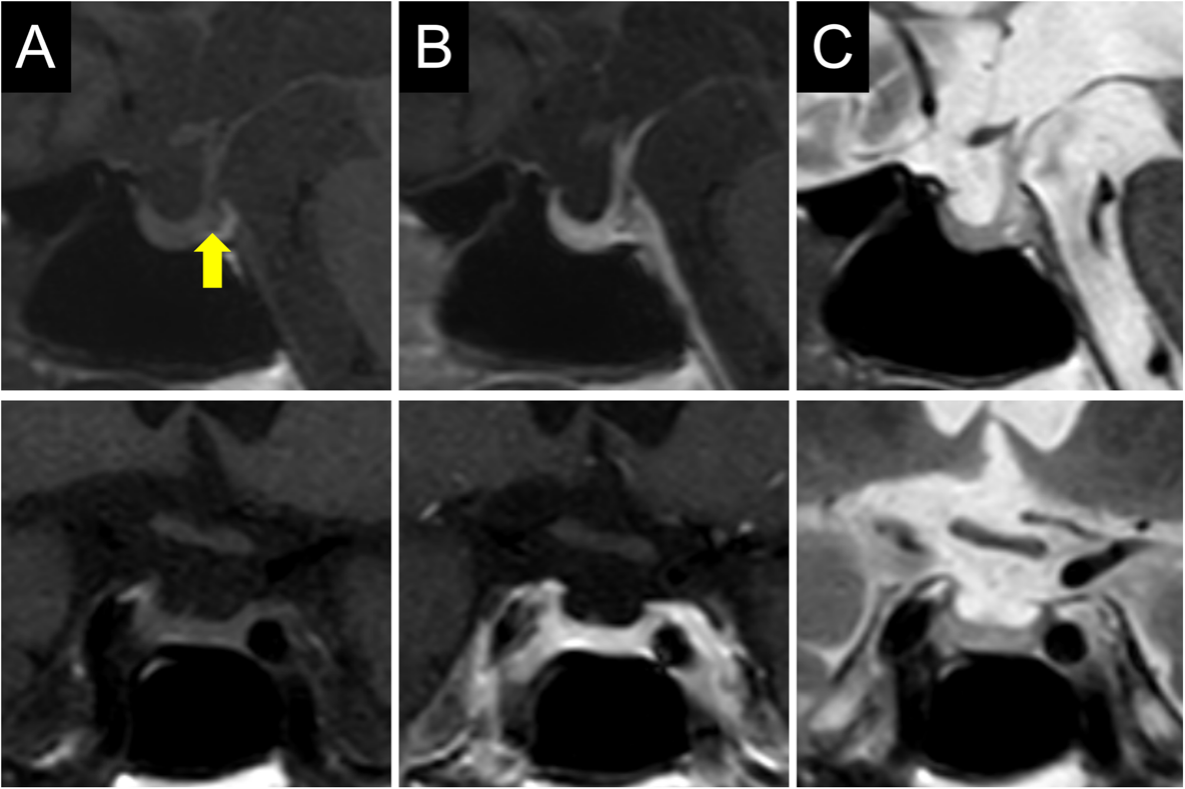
Magnetic resonance imaging of the pituitary. Upper: sagittal plane. Lower: coronal plane. a T1-weighted image; Yellow arrow indicates the loss of pituitary bright spot. b Gadolinium-enhanced image. c T2 weighted image
